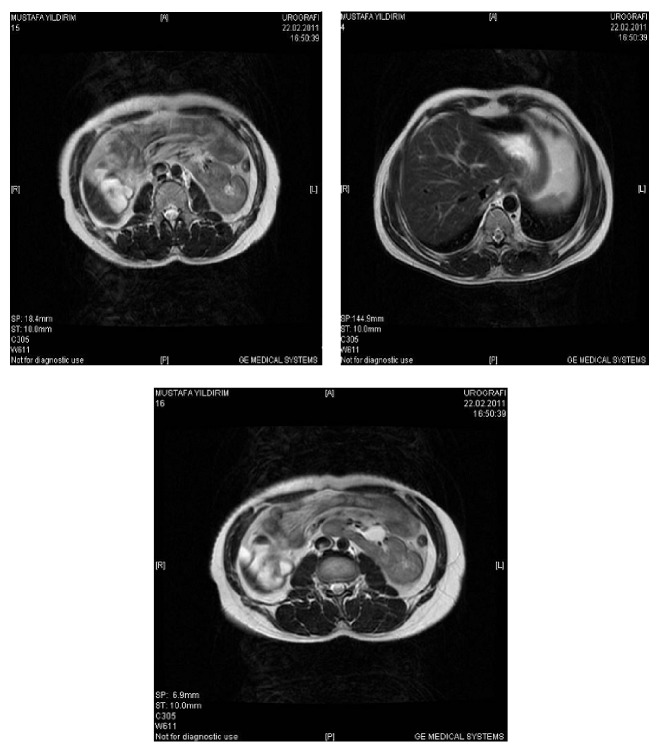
Abdominal CT showing cross ectopy and malrotation of the right kidney.
